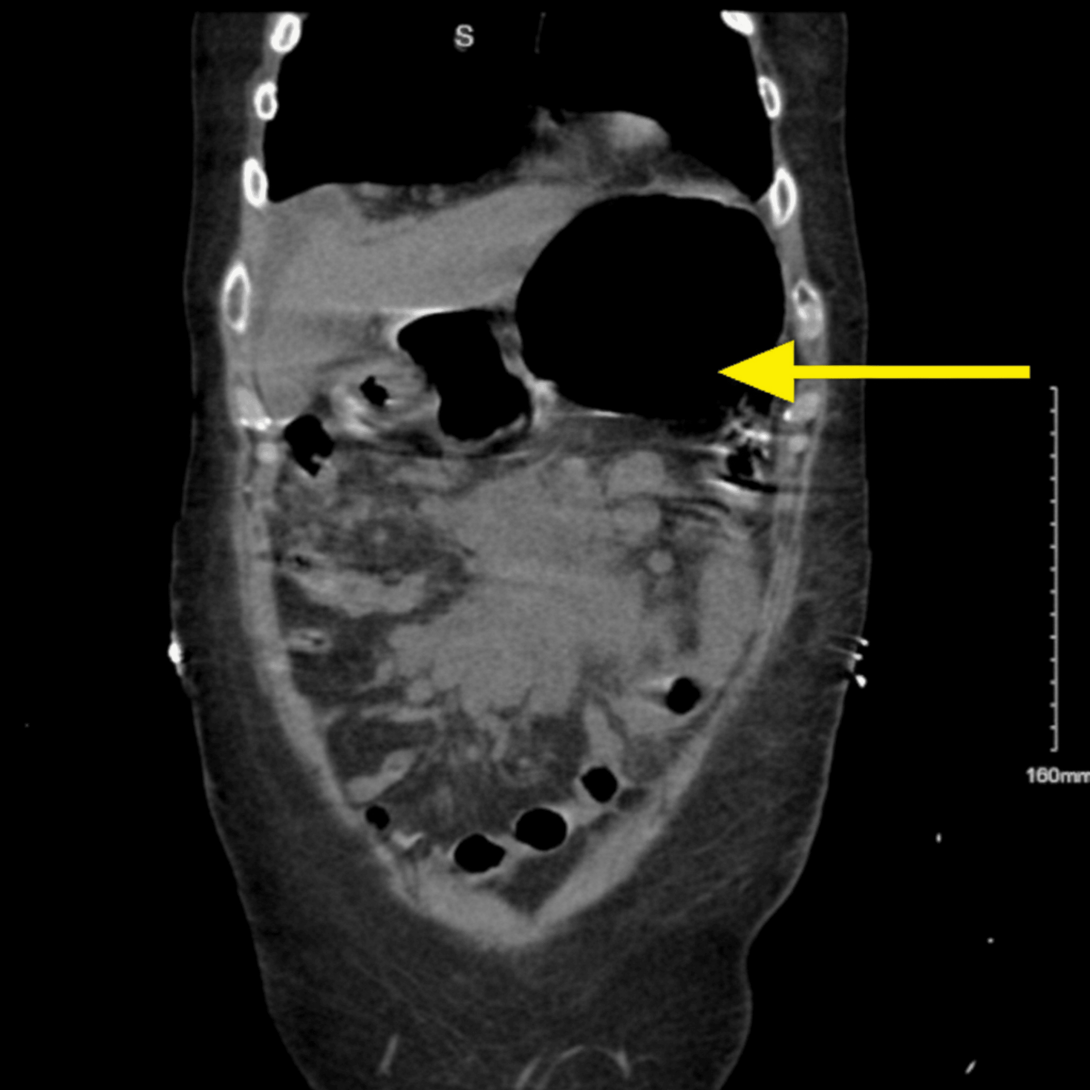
Coronal section of CT abdomen and pelvis showing the gastric dilatation secondary to the gastric outlet obstruction The yellow arrow indicates the dilated stomach from gastric outlet obstruction.
